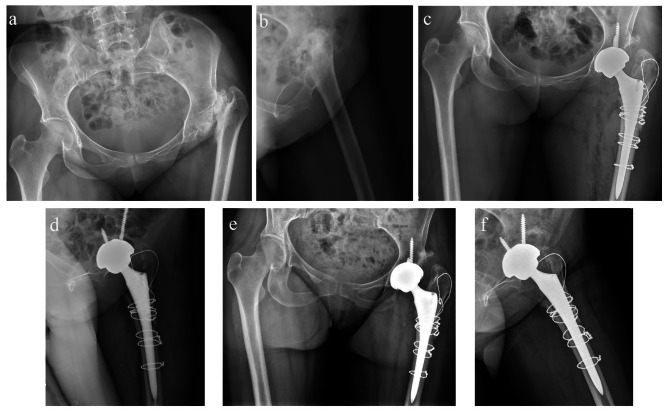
a, b preoperative anteroposterior pelvic radiographs; c, d Hip X-ray after left proximal femur reconstruction + THA; e, f X-ray reexamination 6 months after surgery indicated that the proximal femur had osseous union
